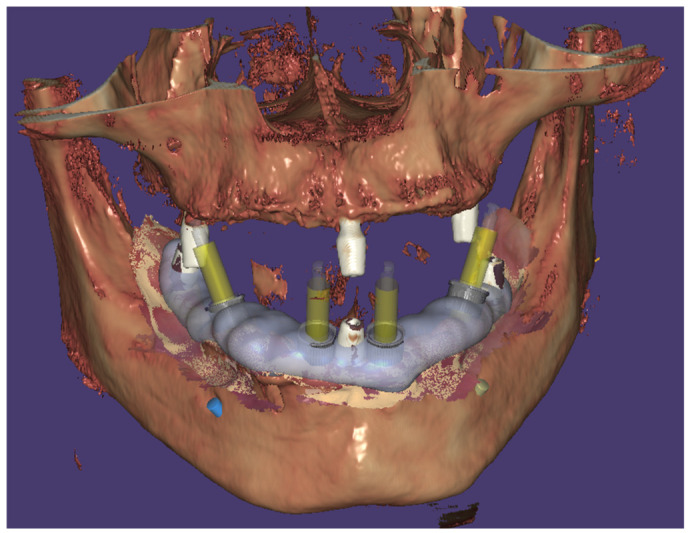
Digital implant planning performed in Exoplan software showing the merged CBCT (DICOM) and intraoral scan (STL) datasets and the planned implant positions within the edentulous arch.
